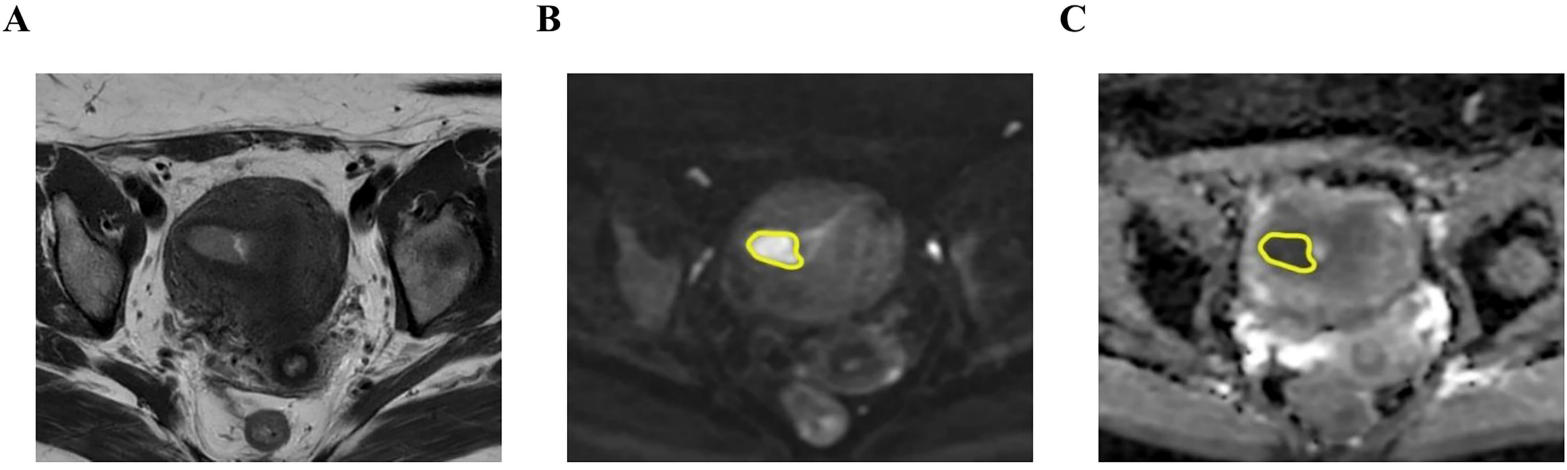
A 52-year-old woman with non-p53 abnormal endometrial cancer (endometrioid carcinoma, G2, stage IA2). The tumor shows slight hyperintensity on axial T2-weighted imaging (T2WI) (A), hyperintensity on axial diffusion-weighted imaging (DWI) (b=1000s/mm^2^) (B), and hypointensity on apparent diffusion coefficient (ADC) map (C). The region of interest (ROI) was drawn along the contour of tumor on DWI and subsequently transferred to the corresponding ADC maps through an automated process (yellow in B, C).
